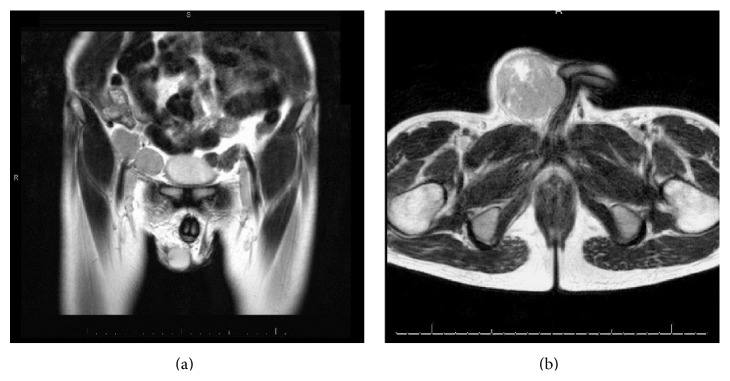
MRI coronal (a) and transverse (b) section showing mass lesion in the right inguinal region with mass effect on the corpora cavernosa of the penis.
